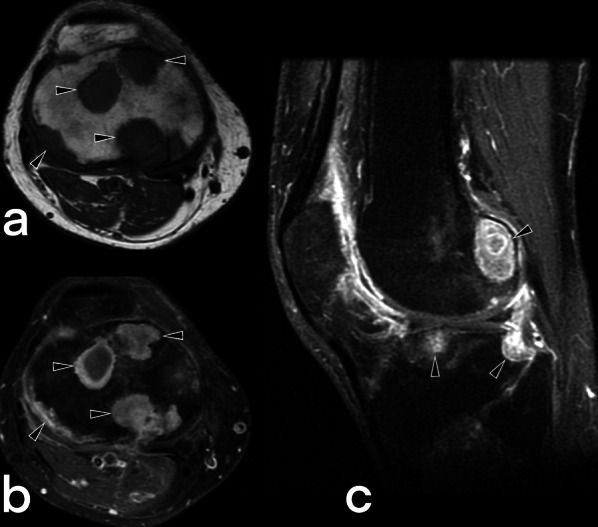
MR images of a 60-year-old male. Pre-contrast axial (a), post-contrast axial (b), and sagittal (c) T1 weighted images reveal intraosseous abscesses (arrowheads) with peripheral enhancement in the distal part of the femur and proximal tibia
